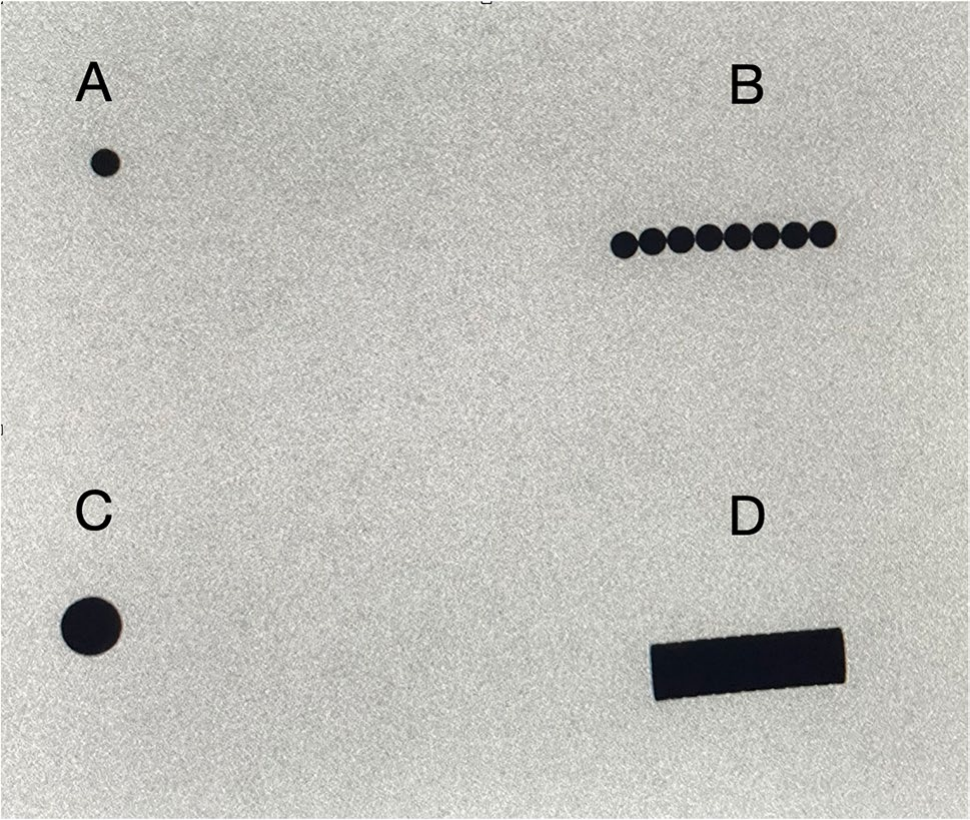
X-ray views of magnets used in this study. Fluoroscopic spot images of (A) single Buckyball magnet, B 8 Buckyball magnets aligned, C single button magnet, and (D) stack of button magnets
